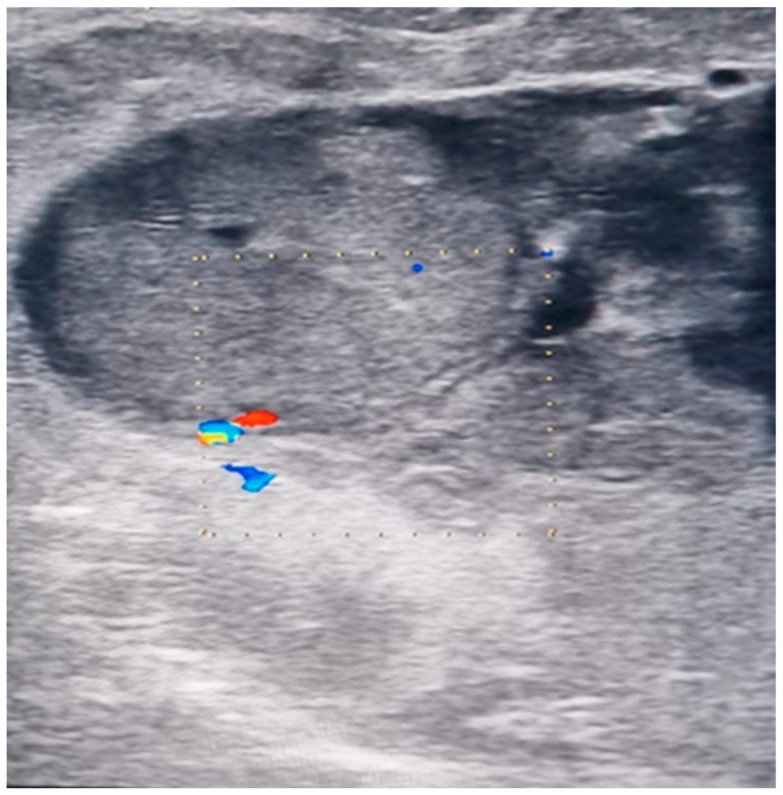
EcoDoppler image of the tumor and vascularization (personal collection).
